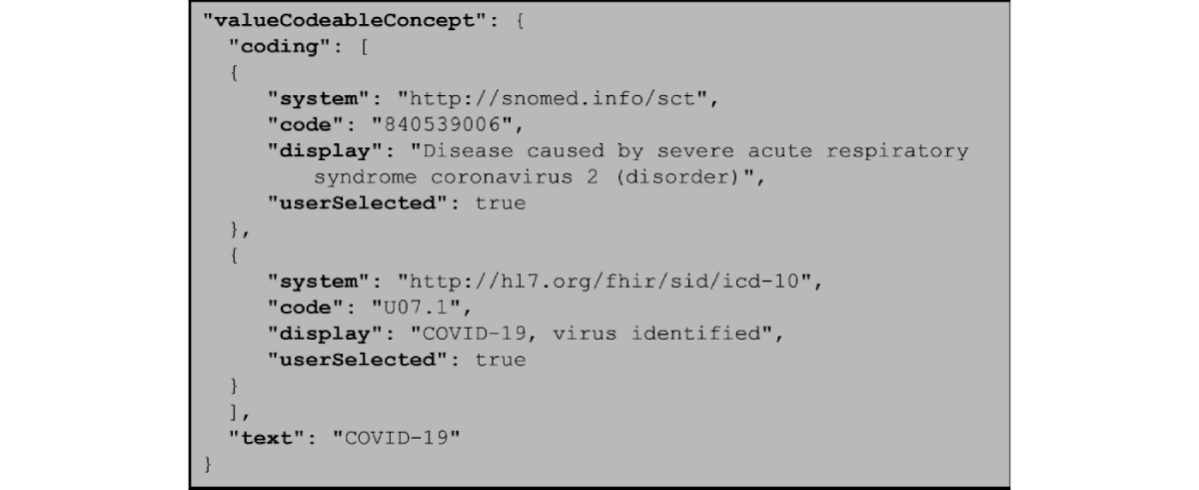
Sample *valueCodeableConcept* element showing multiple *coding* elements. In this example, the *coding* element represents the COVID-19 disease with both SNOMED CT and ICD-10 terminologies. ICD-10: International Classification of Diseases, Tenth Revision; SNOMED CT: Systematized Nomenclature of Medicine—Clinical Terms.
